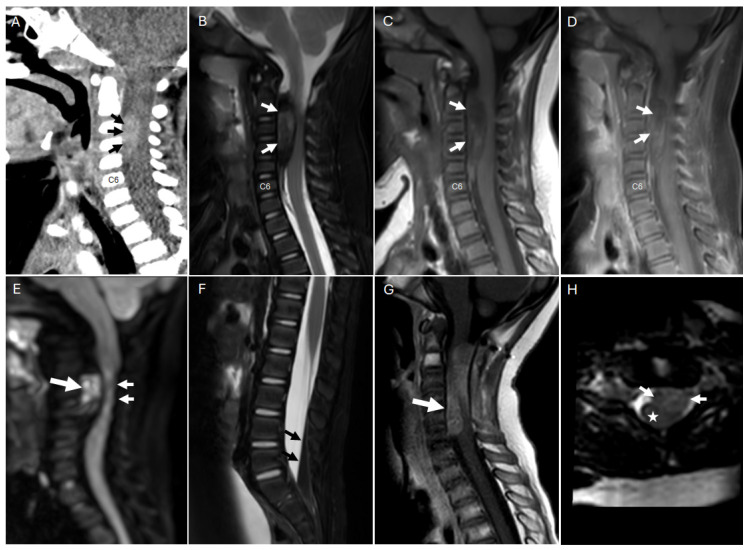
CT and MRI images of a cervical spAT/RT lesion in a 3-year-old patient: (A) Sagittal CT image of the cervical spine demonstrates slightly hyperdense mass in the anterior aspect of the cervical spinal canal (black arrows). (B) Sagittal T2W image demonstrating an intradural, extramedullary mass extending from C2 to C6 (white arrows), appearing heterogeneously isointense and hypointense relative to the spinal cord. (C) Sagittal T1W image demonstrating an intradural, extramedullary mass extending from C2 to C6 (white arrows), appearing heterogeneously isointense and hypointense relative to the spinal cord. (D) Sagittal post enhanced T1W image of the cervical lesion showing poor enhancement following contrast administration (white arrows). (E) Sagittal DWI image demonstrating intralesional restricted diffusion with high DWI signal indicating high cellularity (large arrow) with associated mass effect and posterior displacement of the spinal cord (small arrows). (F) Sagittal T2W image demonstrating layering hemorrhage in the distal spinal canal (black arrows). (G) Sagittal post-enhanced T1W image demonstrating enhancing lesion at the surgical bed surrounding the cord representing tumoral recurrence (white arrow). (H) Axial T2W image demonstrating isointense mass (arrows) displacing the cord posteriorly (star) at relapse.
